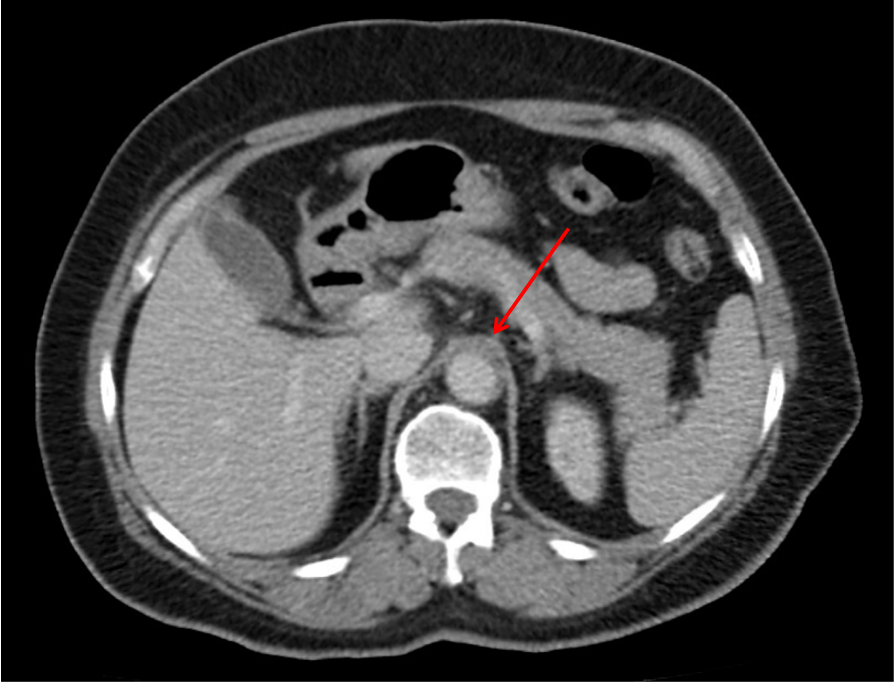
Computed tomography of the abdomen on second presentation revealing mild upper abdominal periaortic stranding with mural thickening of the aorta (*red arrow*), similar in appearance to the left common carotid artery from the original computed tomography of her neck (Fig. 1)
